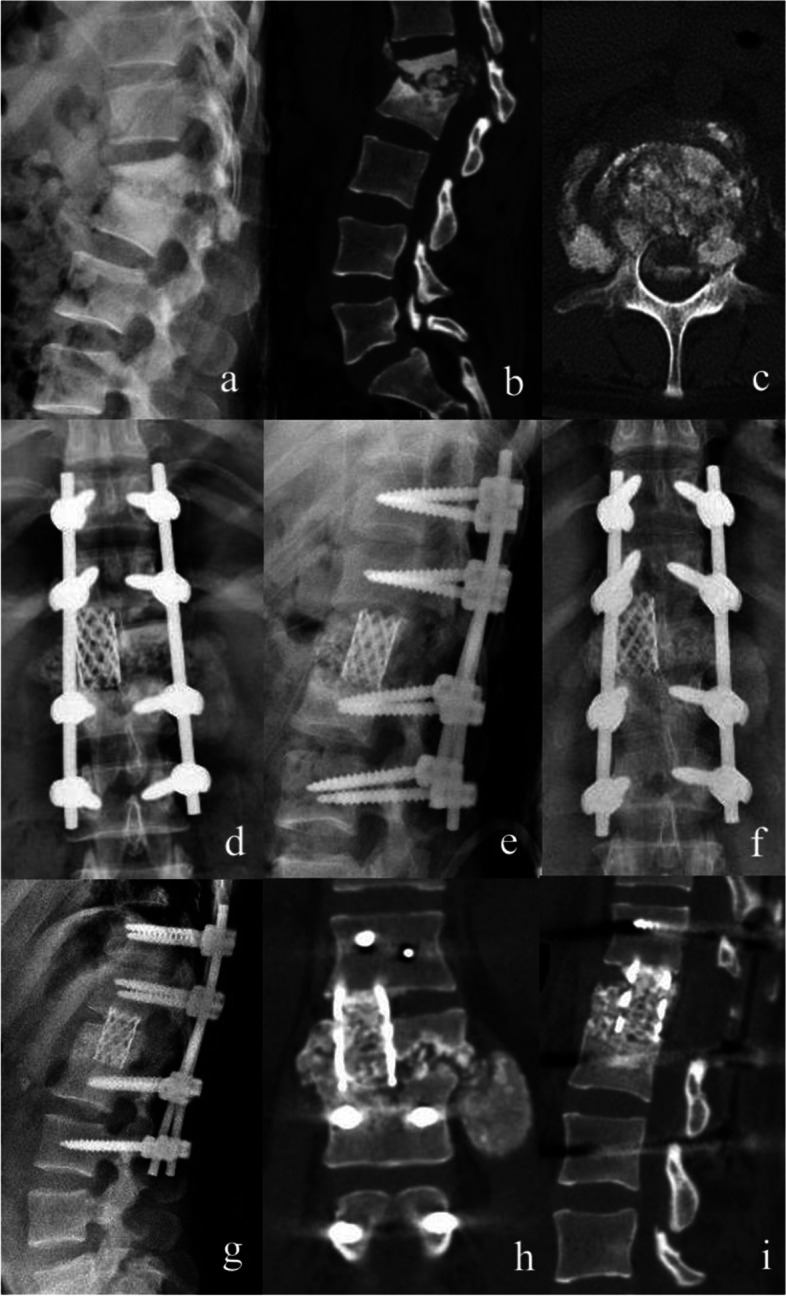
A 31 years old female diagnosed as L1-2 tuberculosis and treated with structural bone graft. a-c Preoperative X-ray and CT scan. d-e Postoperative X-ray. f-i X-ray and CT scan at last follow-up
